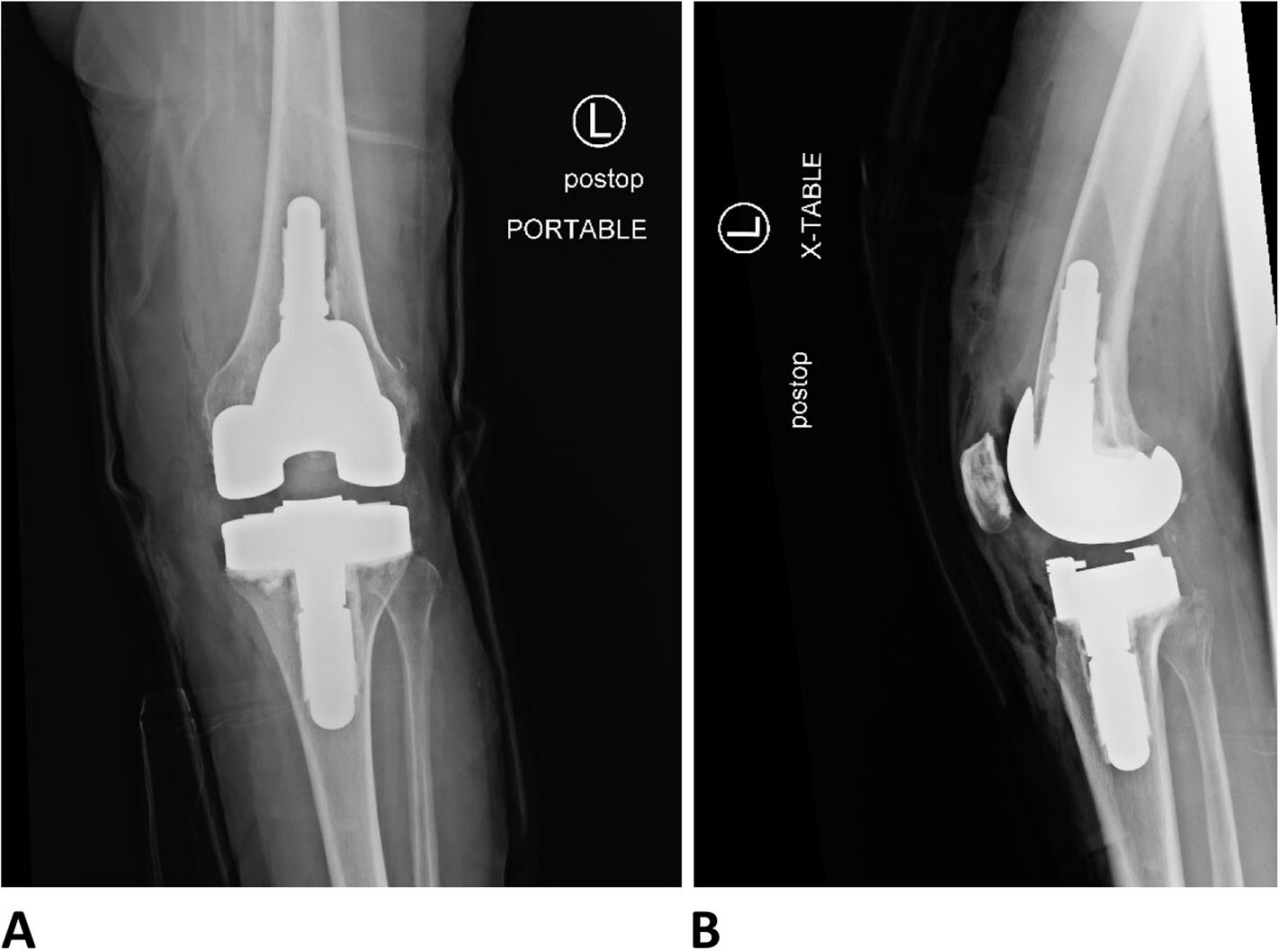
Postoperative radiographs demonstrating successful revision to a total knee arthroplasty using the Zimmer Biomet Vanguard 360 Revision Knee System (a) AP view (b) lateral view
